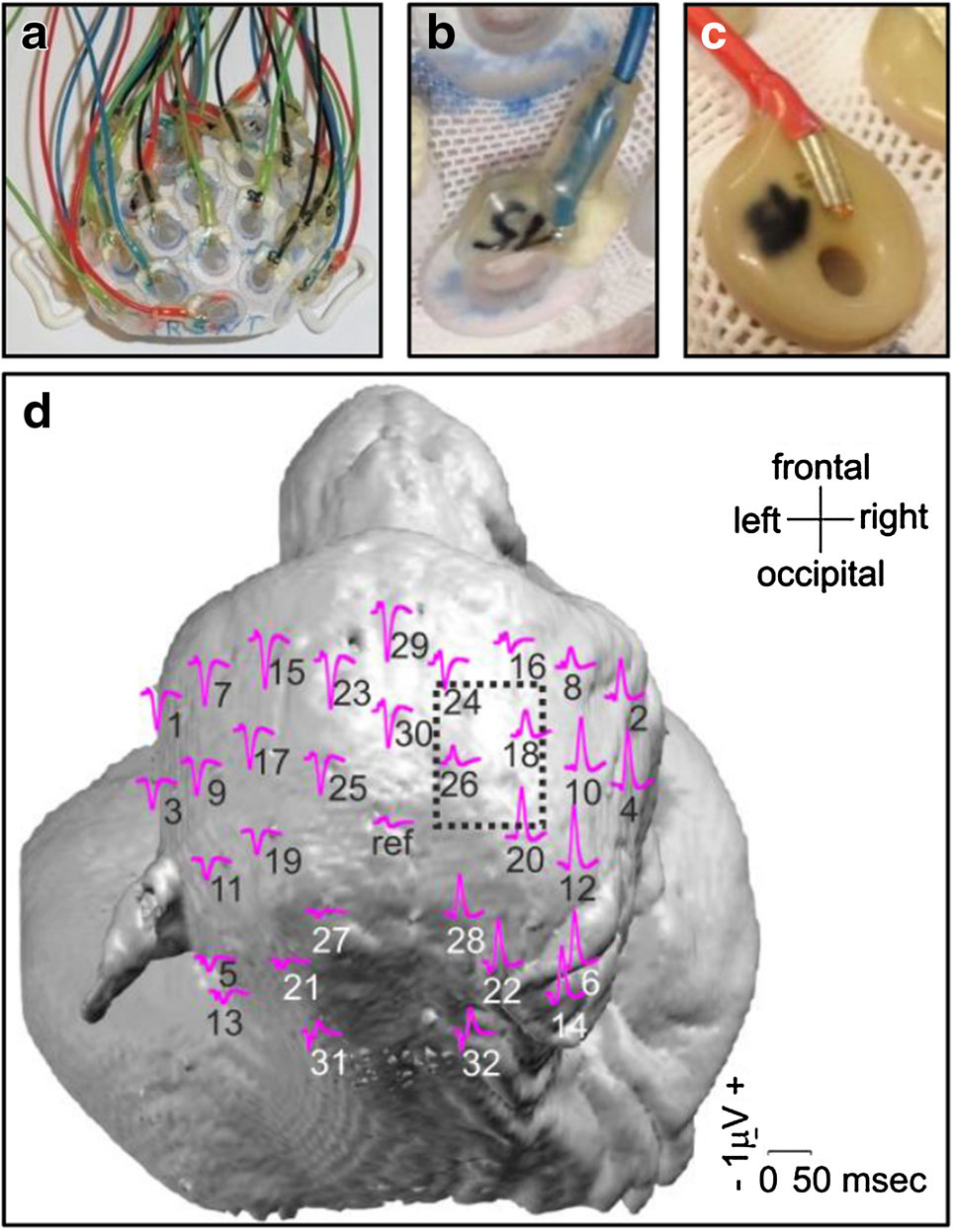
SSEP acquisitions and craniotomy. a View of Mk-EN’s EEG cap from the top (frontal: down, occipital: up, right side: *left*, left side: *right*). b Detail of an electrode of Mk-EN’s cap. The electrode is mounted on a 14-mm circular support which is inserted in the cap. c Detail of an electrode of Mk-AT, Mk-BB and MK-DI’s cap. The electrode is fixed on the cap via a 9.5-mm circular glue support. d Location of the 33 electrodes and their waveform after left median nerve SSEPs (GA of 9 recordings), projected on Mk-EN’s MRI head surface viewed from above (frontal: *up*, occipital: *down*, right side: *right*, left side: *left*). Interval: 0.0 to 50.0 ms post-stimulus. The perimeter of the bone flap of the craniotomy is indicated by *dotted lines*. *ref* reference electrode
